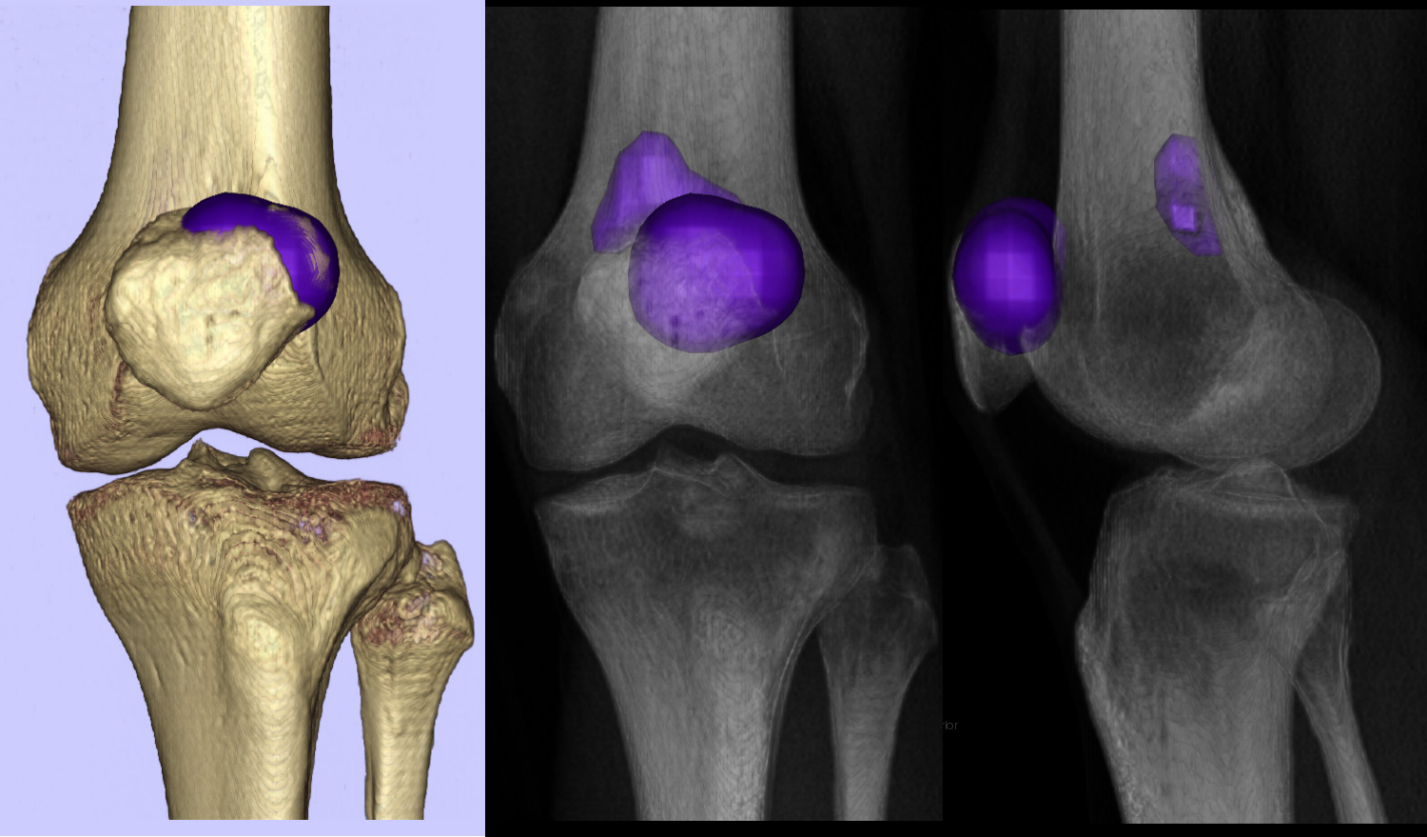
Rendering SPECT region of interest. Once a clinically relevant SPECT intensity threshold has been established, we can render a hotspot surface at that threshold for a visual appreciation of the hotspot size and position.
